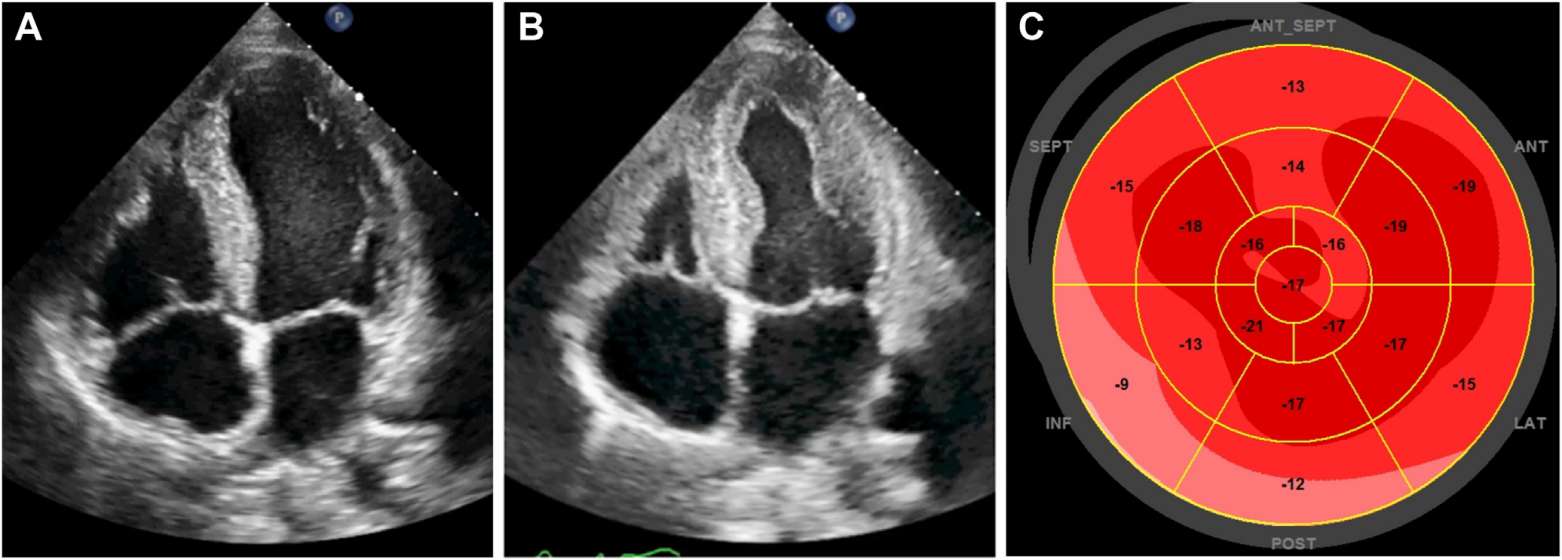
Echocardiographic apical 4-chamber view in diastole (A) and systole (B). Maximal wall thickness was 12 mm, and left ventricular ejection fraction was 53%. Speckle-tracking imaging showed a moderate reduction in left ventricular longitudinal function, with a global longitudinal strain of –14.6%. The reduction in longitudinal shortening was most prominent at the left ventricular base (C).
